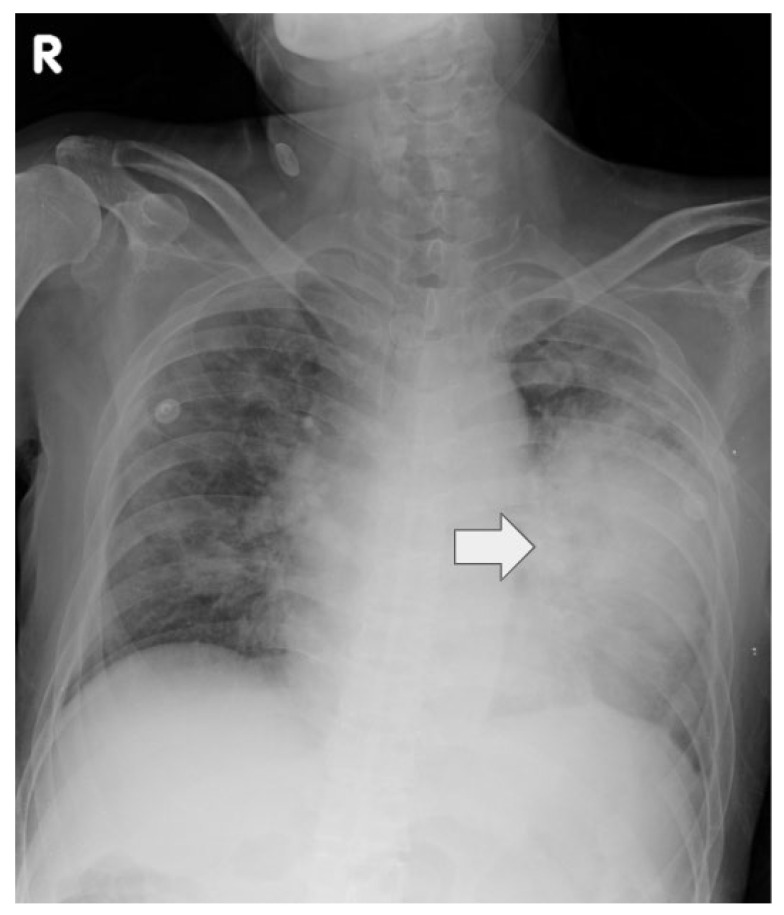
The chest X-ray obtained on admission (16 December 2023) demonstrates bilateral pulmonary infiltrates, with consolidation most prominent in the left lower lung (indicated by the arrow). The endotracheal tube is appropriately positioned. No pleural effusion is seen.
